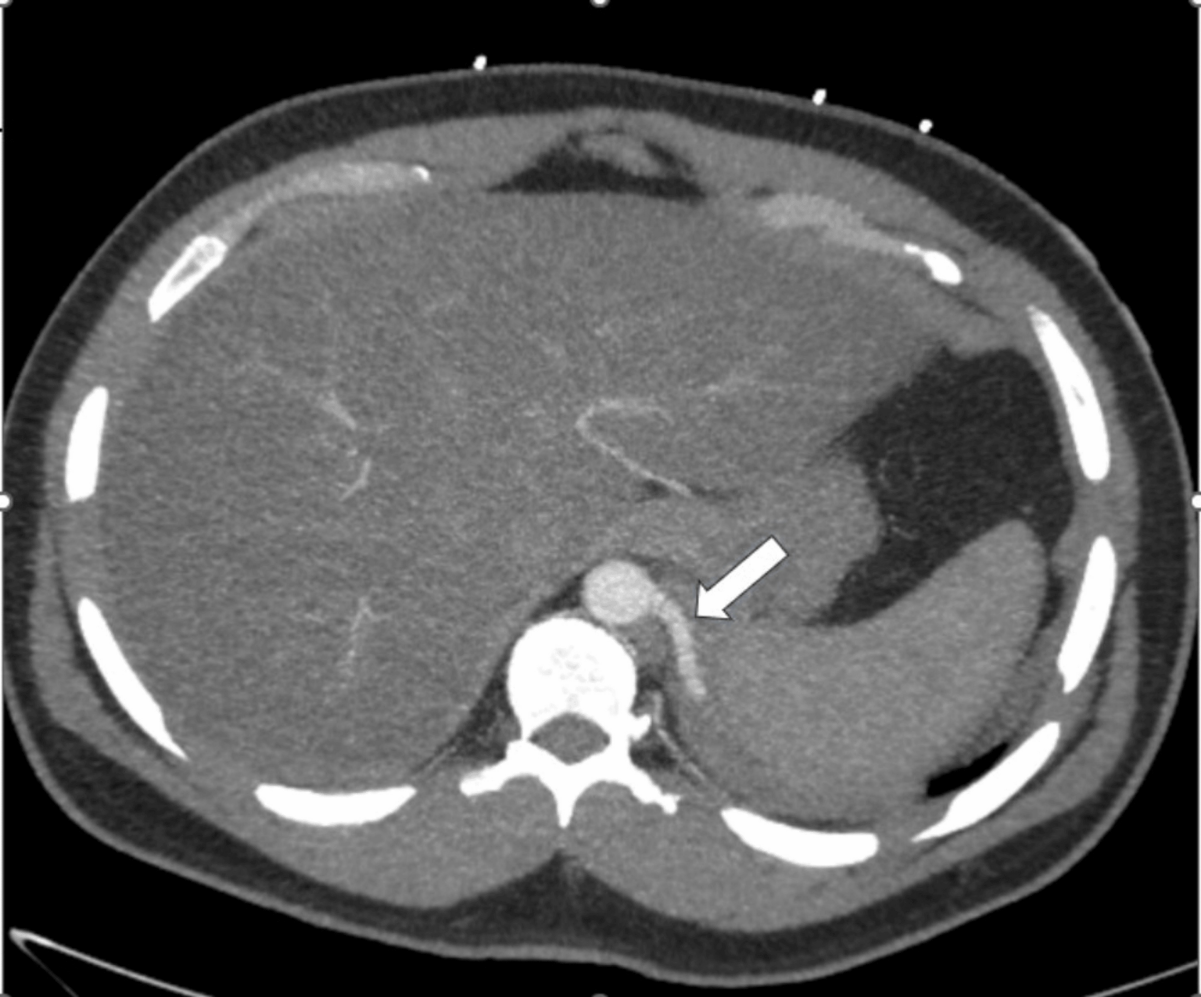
Admission chest CT scan Axial contrast enhanced pulmonary CT angiogram shows an aberrant artery arising from the distal descending thoracic aorta.
